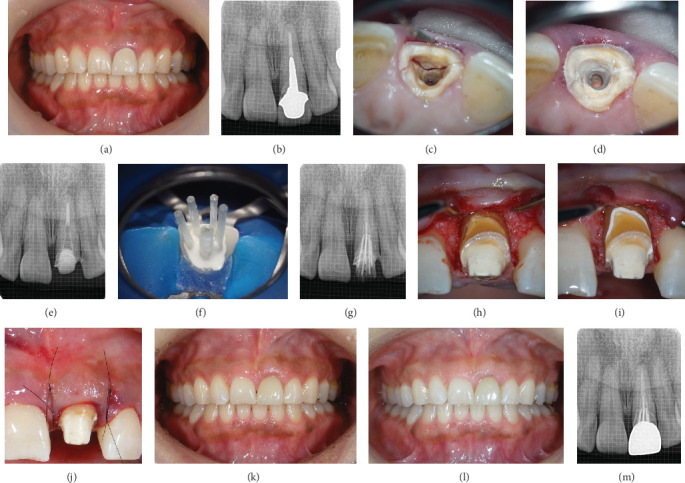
Case 1. (a) Frontal view at the first visit. (b) Radiograph of the anterior teeth taken on the day of the first visit. A translucent image is observed at the cervical area of the maxillary left central incisor. (c) Labial fracture line of the maxillary left central incisor after the removal of the post material. (d) The fractured piece bonded to the tooth root substance. (e) Radiograph after root canal treatment of the maxillary left central incisor. (f) Glass fiber posts distinctively positioned at the center (one main point) and cavity side (four subpoints). (g) Radiograph after foundation construction. (h) The fracture line is visible after opening the flap. (i) The fracture line is covered with MMA-based resin cement. (j) Periodontal tissue after surgery. (k) Frontal view after cementation of the suprastructure. (l) Frontal view 4 years after intraoral cementation. (m) Radiograph 4 years after intraoral cementation.
